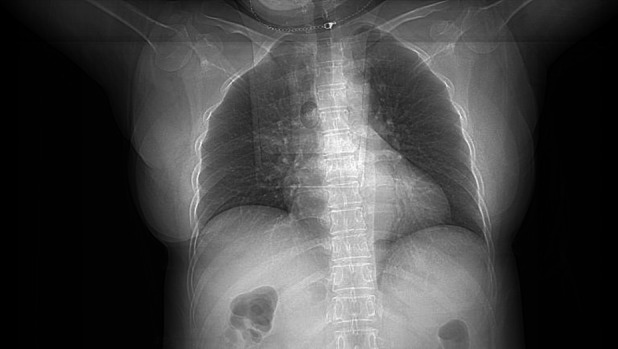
Chest radiograph (CXR) performed on March 24, 2025 showed no obvious abnormalities and no visible infiltrates, cavities, or masses.
